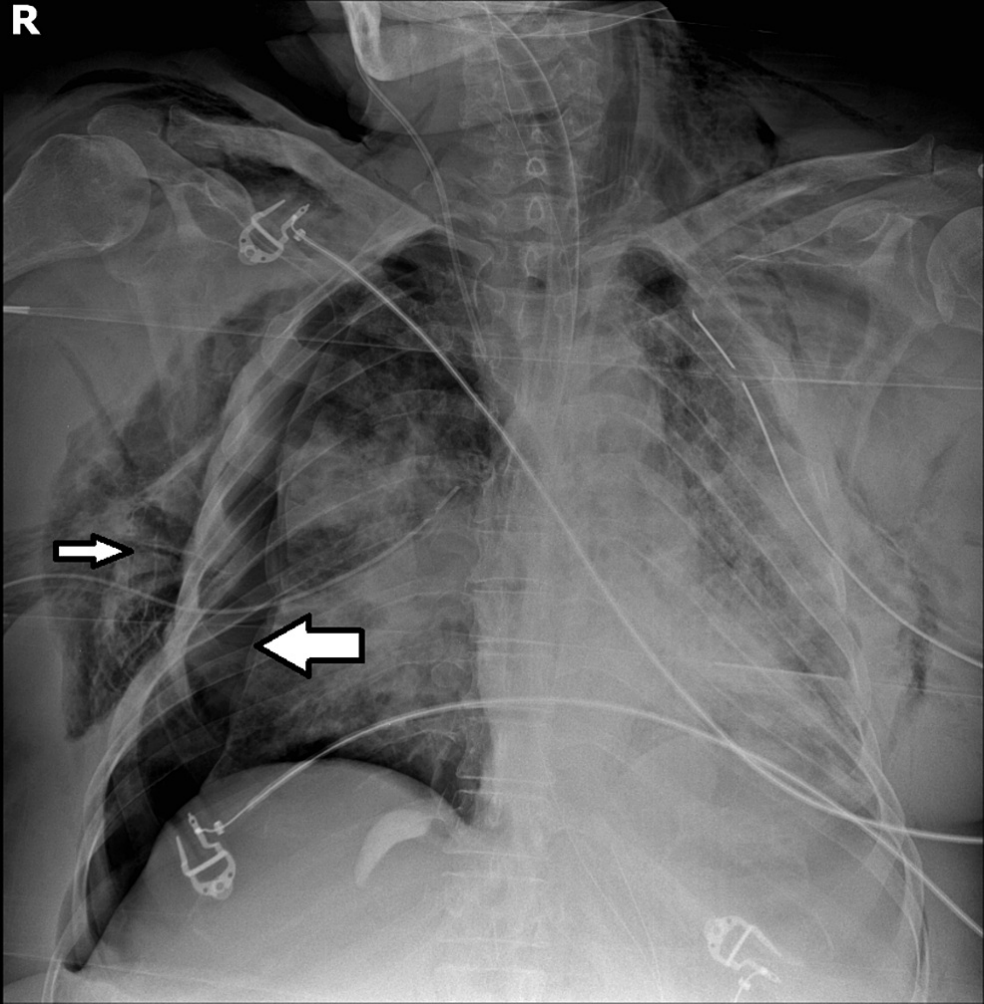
Radiograph of the thorax on the 16th day in the intensive care unit Right pneumothorax (big arrow) and subcutaneous emphysema (little arrow) are evident.
